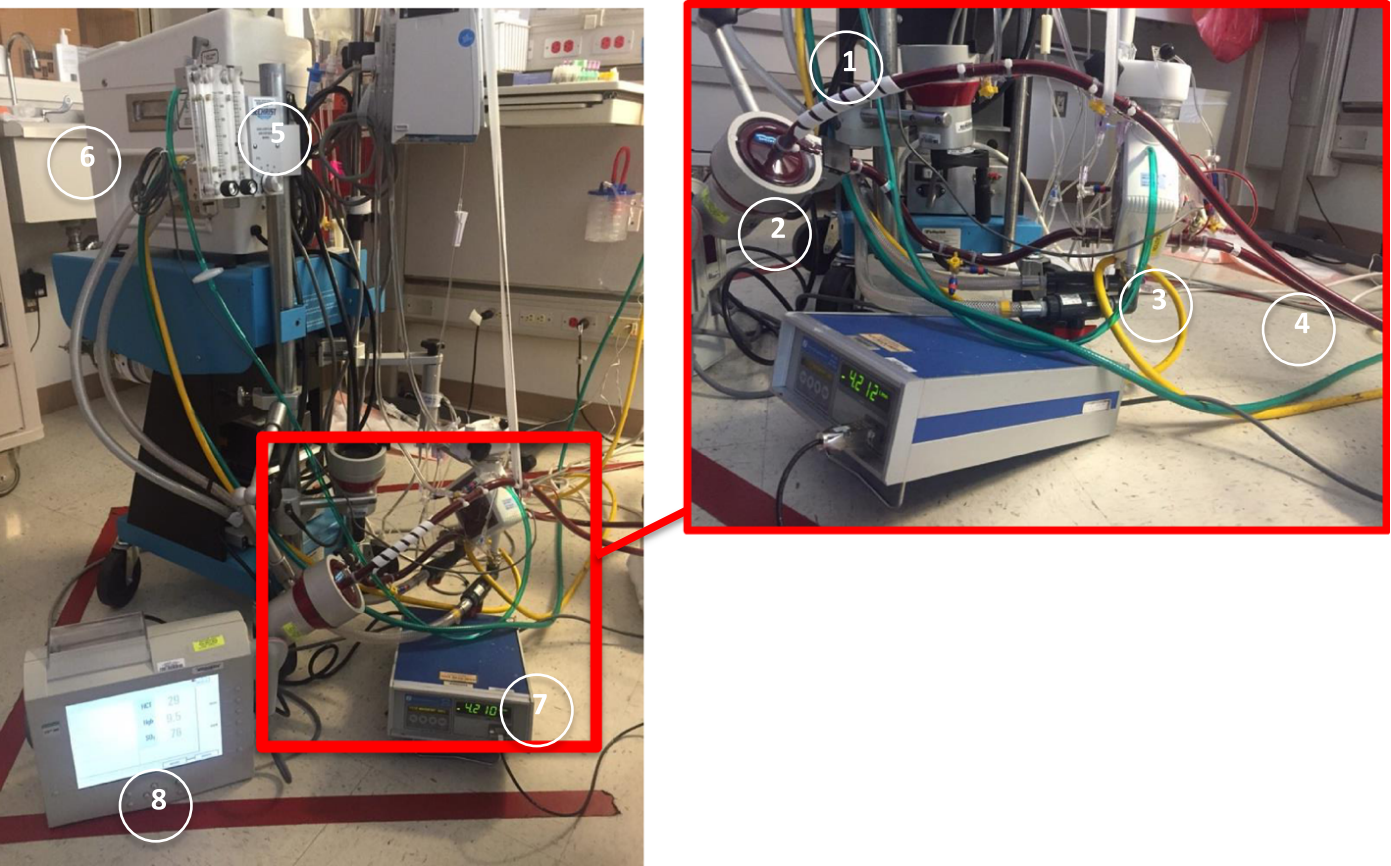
ECMO circuit components and flow. Flow through the ECMO circuit starts with the venous drainage cannula (1), which is propelled forward by the pressure gradient generated by the centrifugal pump head (2). The blood passes through the oxygenator (3) and then returns to the patient in the outflow tubing back into the right atrium (VV) or femoral artery (VA) (4). Gas exchange is regulated by the amount of countercurrent “sweep” gas flowing through the oxygenator (5) and the blood is warmed by the thermoregulator attached to the circuit (6). Flow, hemoglobin, hematocrit and venous saturation can be continuously monitored by ultrasonic meters attached to the circuit (7 and 8)
